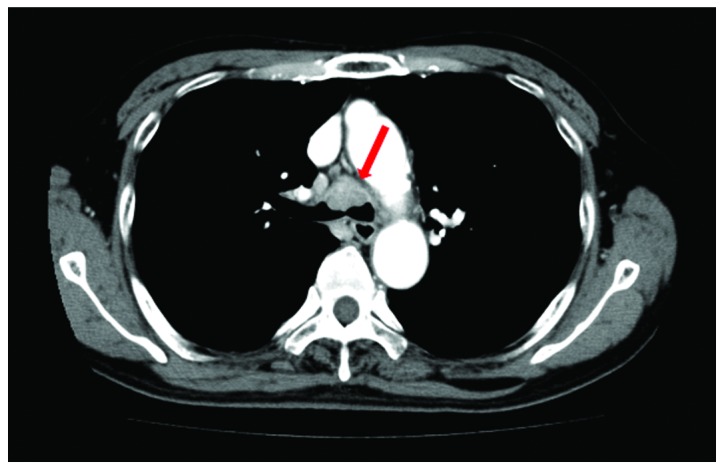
Chest computed tomography scan. The tumor measured 25×20×15 mm and was located directly above the carina and protruded from the anterior wall of the trachea (arrow).
